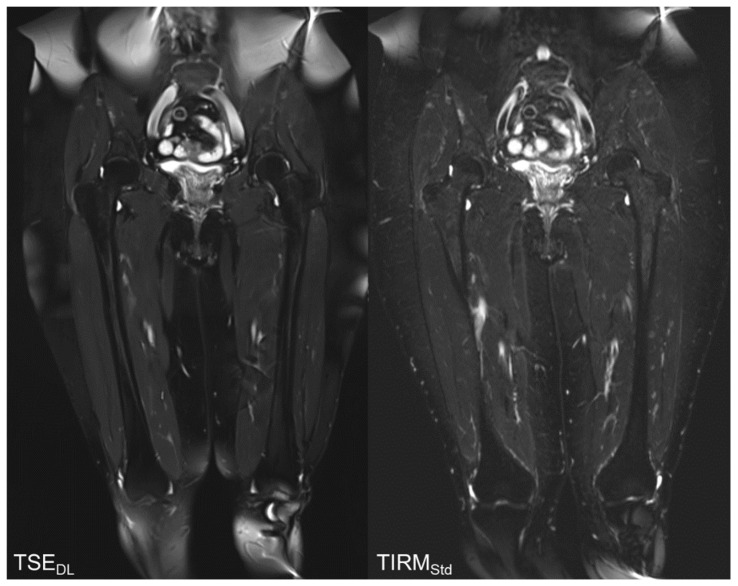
Follow-up MRI examination of an 81-year-old patient with Merkel cell carcinoma. As an incidental finding, the coronal TIRM_Std_ sequence shows a lipoma of the medial vastus muscle. The T2w TSE_DL_ shows a better image quality, noise, and sharpness than the TIRM_Std_.
